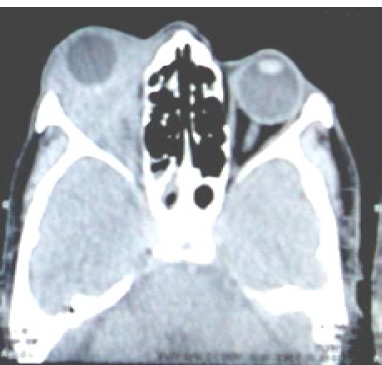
CT ORBIT - Plain and with contrast - Homogenous isodense lesion observed without any distortion of the globe
